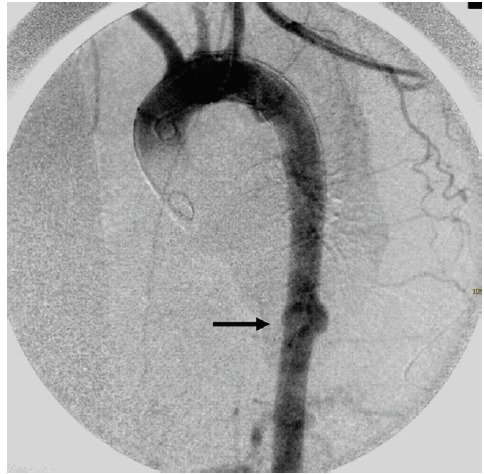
No signs of endoleak were detected by the second post-procedural angiography (Figure 3a) and by CT scan performed after a week (Figure 3b).
